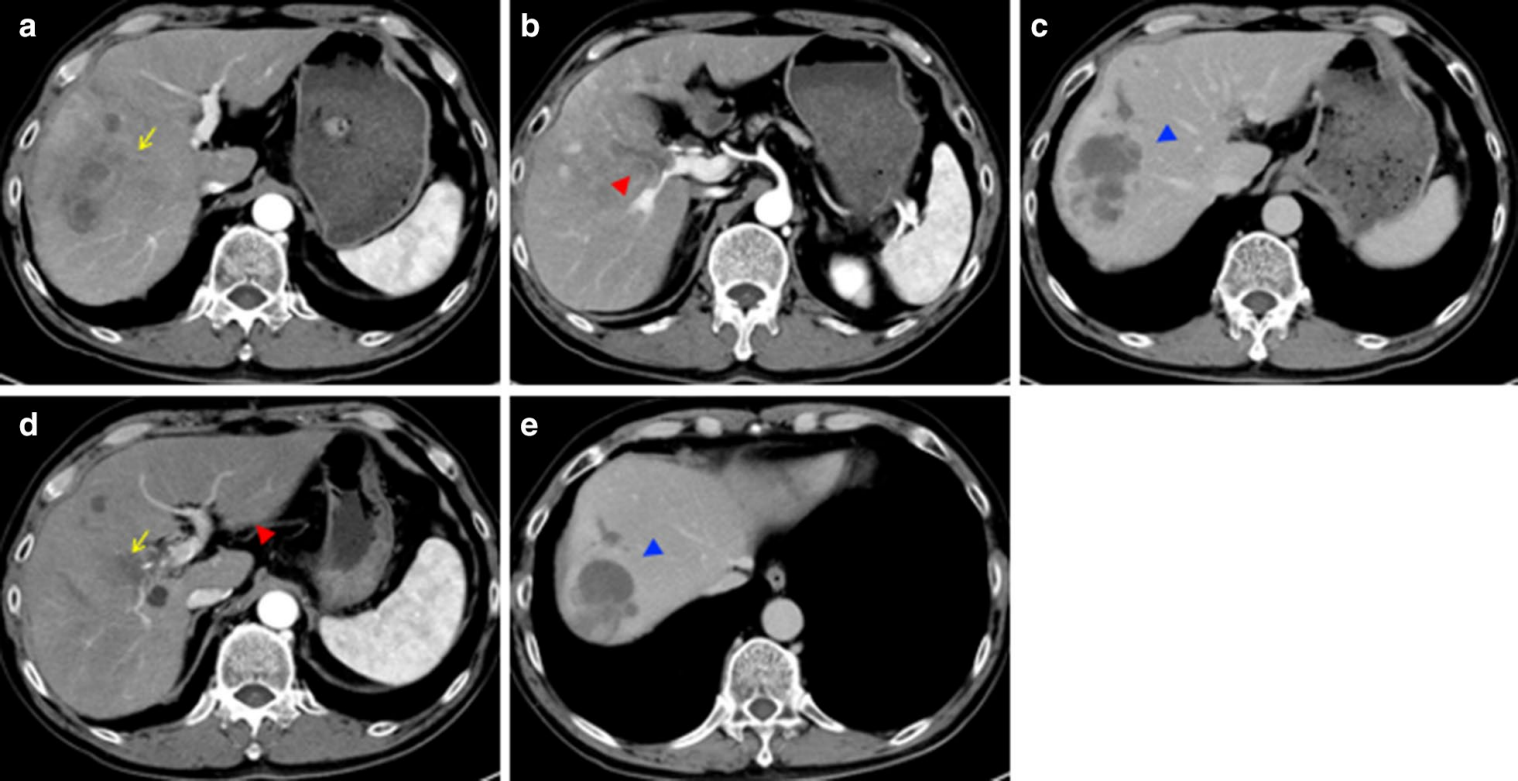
Abdominal enhanced CT findings in the course. a–c After two weeks of lenvatinib administration before the portal vein transection. CT showed reduction of tumor vascularity (yellow arrow), and extension of portal vein invasion (red arrowhead), as well as tumor necrosis (red arrowhead). d, e One month after the right portal vein transection. Absent flow in the right portal vein (yellow arrow), enlargement of the left lateral segment (red arrowhead), and progression of tumor necrosis (blue arrowhead)
